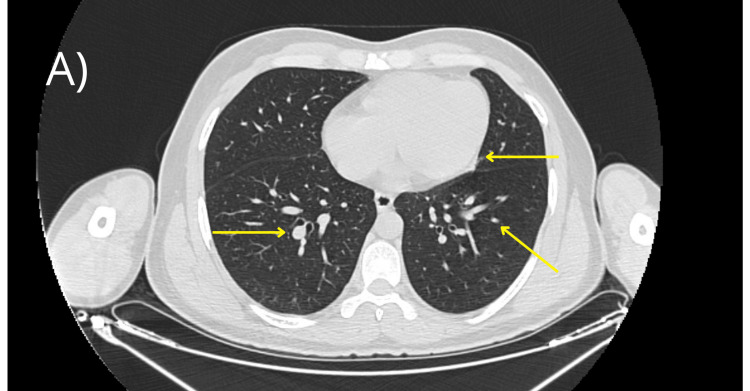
Follow-up contrast-enhanced thoracic CT showing decreased size of pulmonary lesions.
